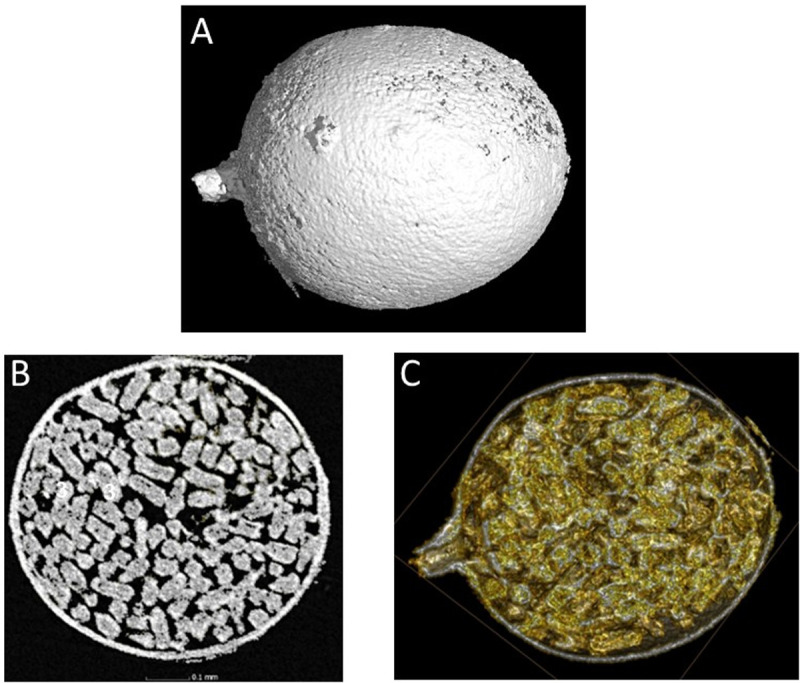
Xray Computed Tomography scanning of a *Globodera pallida* cyst. *G. pallida* cysts were scanned via X-ray CT to visualise the cuticle (A) and interior contents from 360^0^ (B, C). Cross-sectional photos at 50% depth show distinct egg profiles (B, C).
